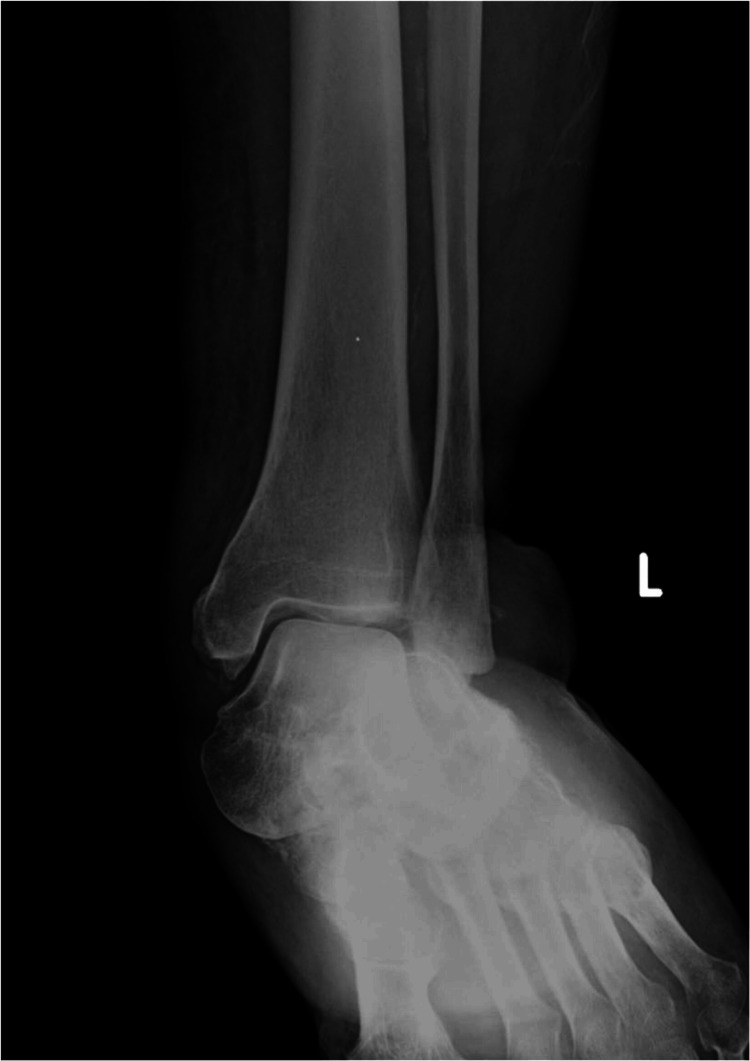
Preoperative anteroposterior ankle X-ray
